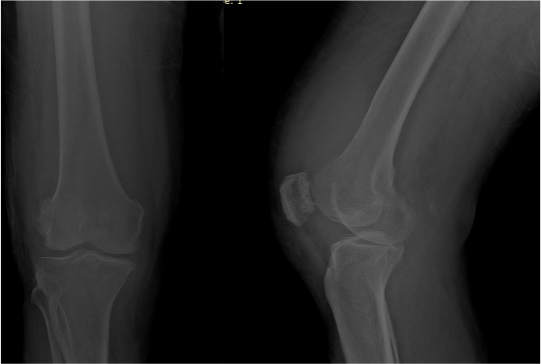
Radiographic findings after the removal of large loose body. Anteroposterior (a) and lateral (b) X-ray of the knee
